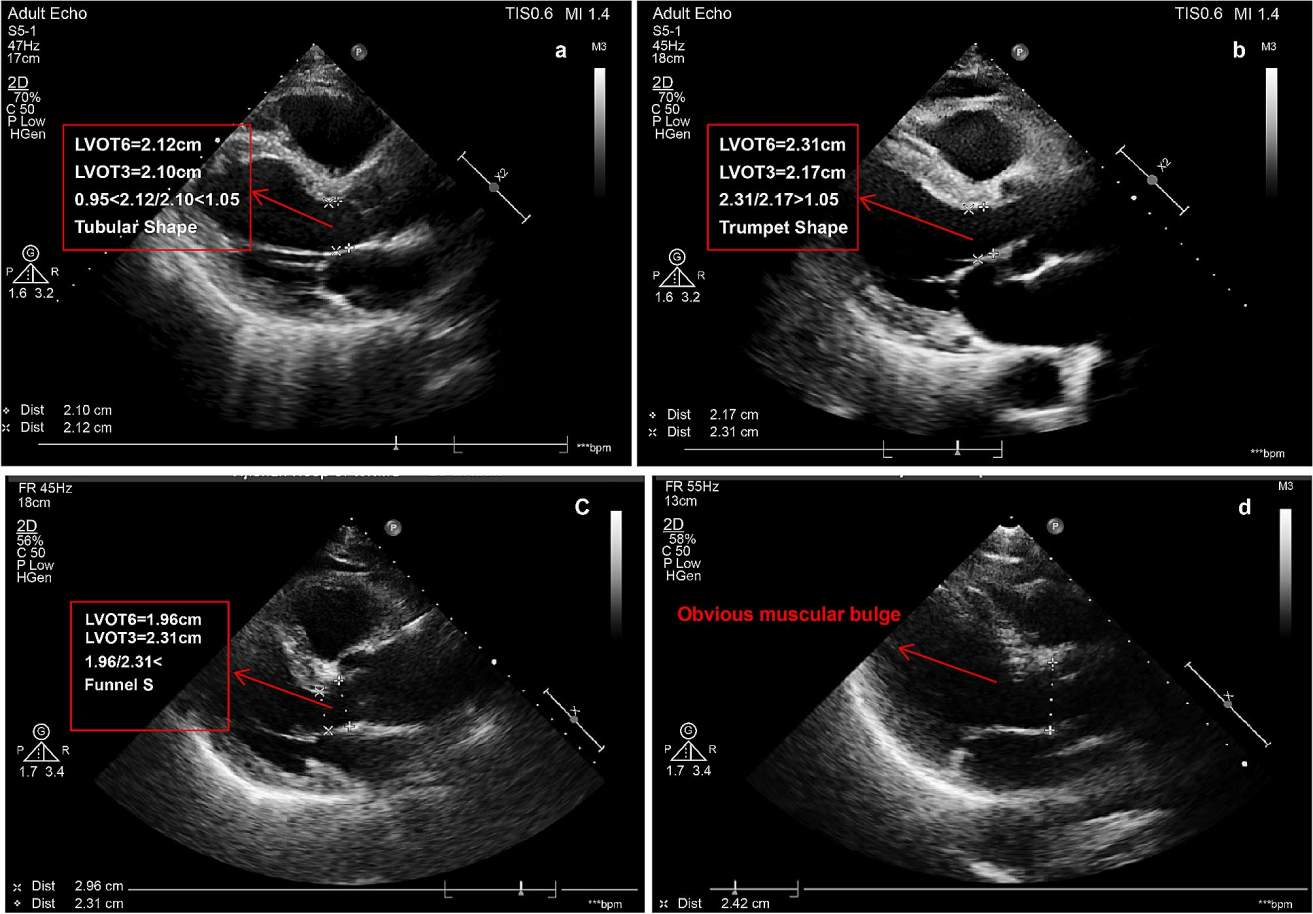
LVOT morphology detected by TTE (a) LVOT shape is tubular; (b) LVOT shape is like a trumpet; (c) LVOT shape is like a funnel; (d) LVOT shape is also like a funnel, as there is an obvious muscular bulge in the inner ventricular septum 6 mm below the aortic valve annulus. Here, the trumpet and funnel shapes were classified as non-tubular shapes; therefore, the LVOT in b, c, and d are all referred to as non-tubular shapes LVOT: ventricular outflow tract; TTE: transthoracic echocardiography
